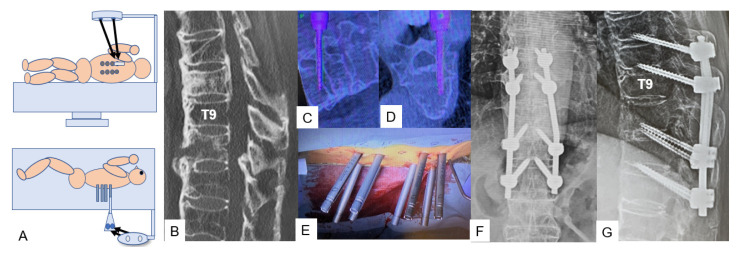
Case 2, 82-year-old male, DISH, T10 fracture, Lateral Bendini. (A) Bendini image, (B) Preoperative sagittal reconstruction CT, (C,D) Navigation monitor, (E) Intraoperative image, (F) Postoperative anteroposterior radiogram, (G) Postoperative lateral radiogram.
